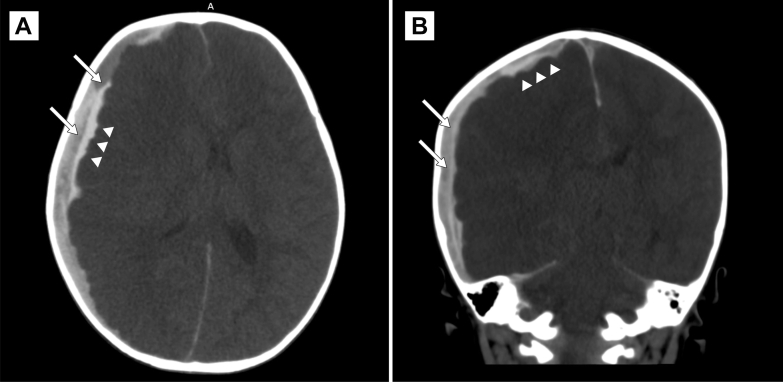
Noncontrast Computed Tomography of the Brain (3 Hours Post Procedure) (A) Axial plane. (B) Coronal plane. Large hypodense area in the right hemisphere consistent with acute infarction/ischemia, with associated mass effect. Two layers of mixed-density crescent-shaped collection along the right cerebral convexity likely represent contrast accumulation (arrowheads) and subdural hematoma/fluid (arrows).
